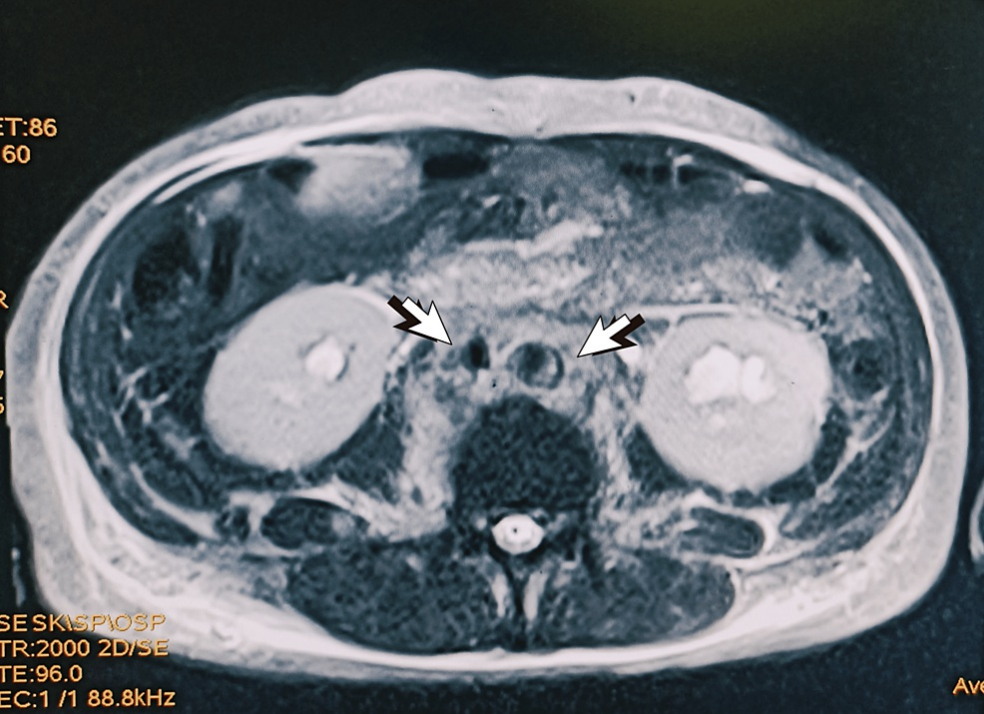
Axial T2W image of the abdomen showing heterogenous soft tissue encasing IVC and abdominal aorta (white arrows) T2W: T2-weighted image, IVC: inferior vena cava.
